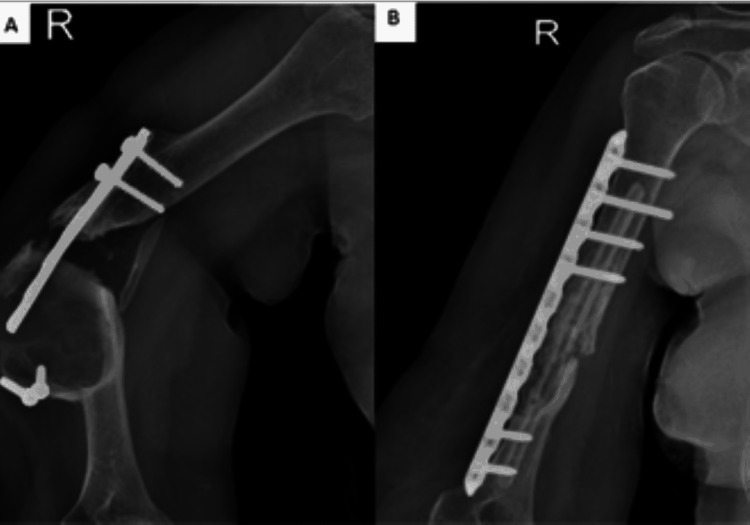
(A) Radiographs demonstrating non-union of the humerus after failed osteosynthesis. (B) Postoperative radiograph demonstrating healed fracture with locking compression plate and fibular graft.
